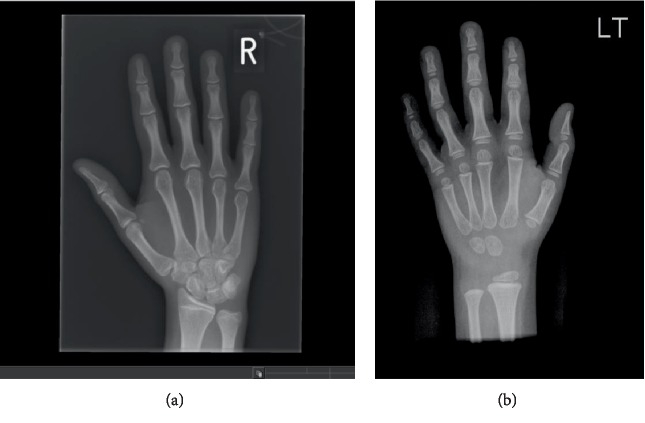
(a and b) B1 radiograph of right hand aged 15 years (a). B1 radiograph of right hand aged 3 years (b).
